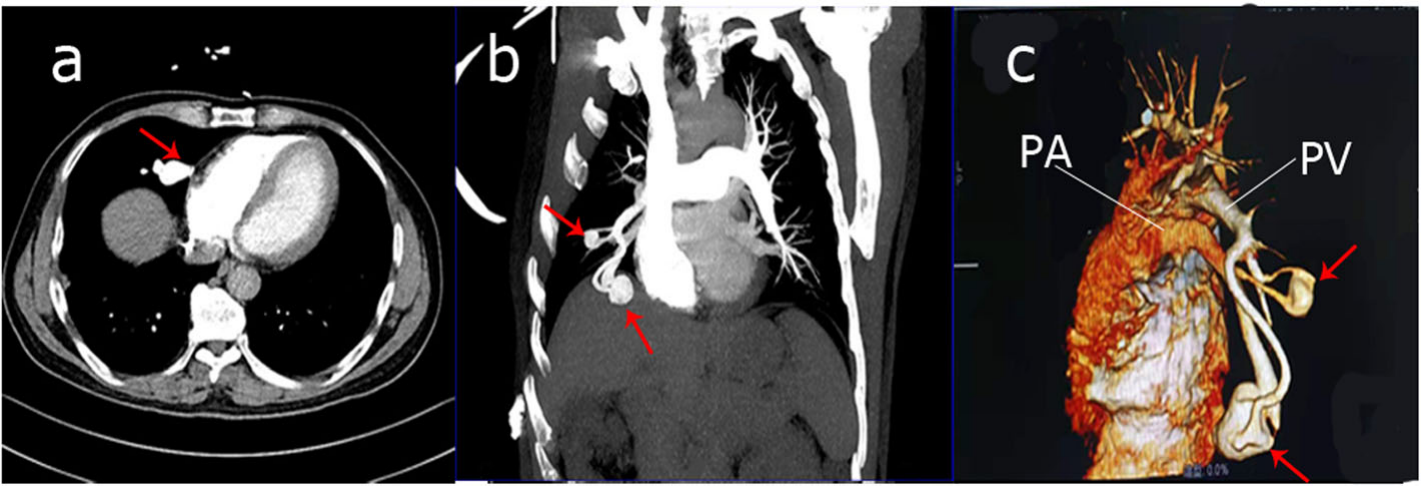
Pulmonary CTA findings. Axial thoracic CTA shows two irregular high-density nodules in the middle of right lung, maximum cross section 2.1 cm × 2.1 cm (a); (b) MIP and (c) VRT confirm PAVFs (red arrow). CTA, computed tomography angiography; MIP, maximum intensity projection; VRT, volumetric reproduction technique; PA, pulmonary artery; PV, pulmonary venous
